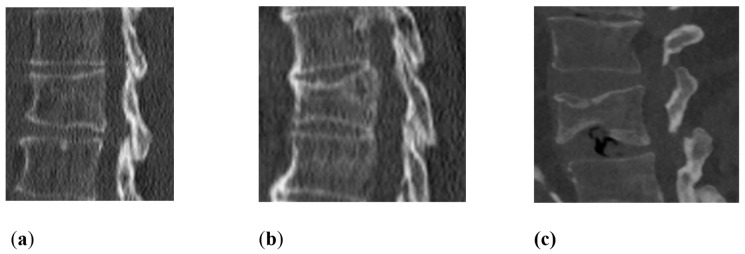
Genant score in sagittal CT scans: (a) Grade 1 20–25% height loss. (b) Grade 2 26–40% height loss. (c) Grade 3 > 40 %.
